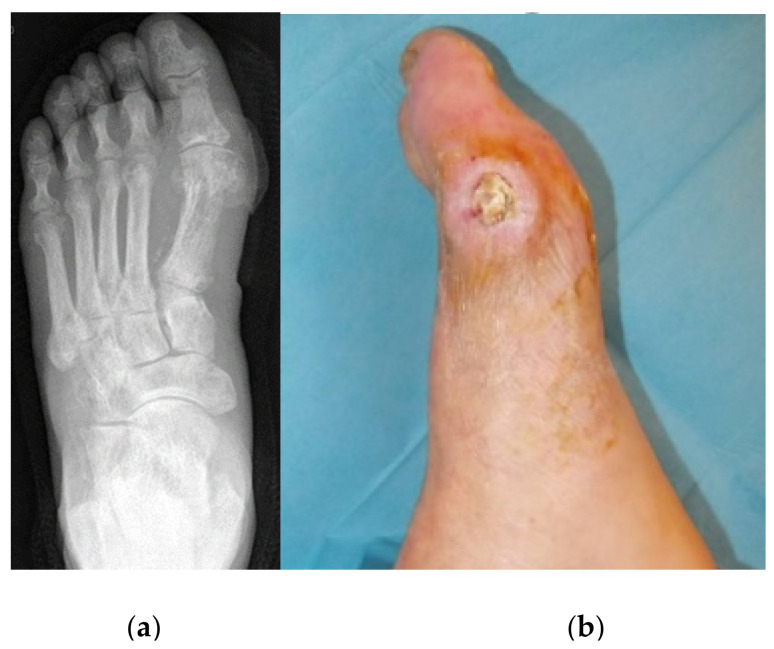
Pre-operative X-ray showing osteomyelitis of the first metatarsal head (a), and pre-operative and photograph of the lesion (b).
